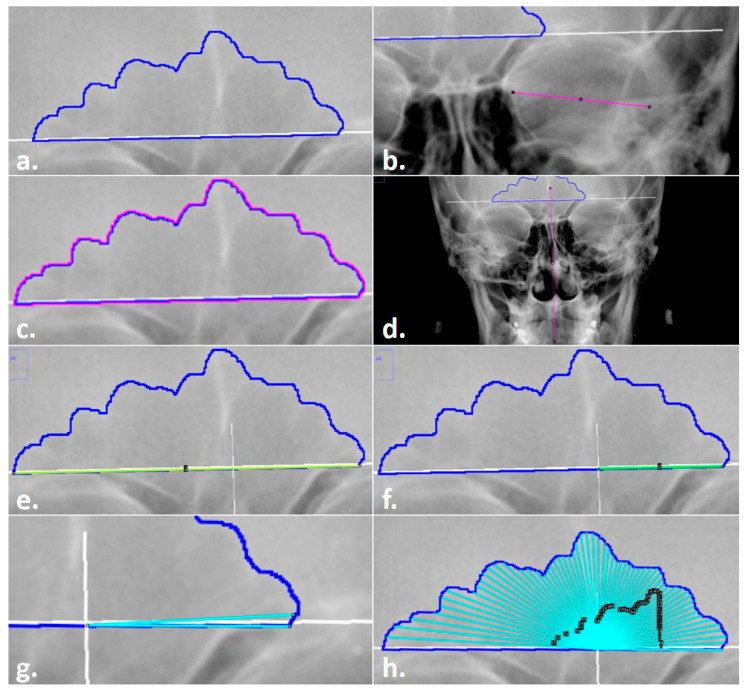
Steps in the Total Difference Method: (a) sinus outline on a radiograph; (b) measurement of orbital breadth for scaling; (c) highlighted sinus outline to be measured for the area and perimeter; (d) line drawn through midline landmarks to identify the origin of the sinus baseline; (e) line drawn for the baseline measurement; (f) line drawn from the origin for the right (side of the image) baseline measurement; (g) preliminary angle drawn at 3°; (h) completed angles with black ROI Manager numbered labels for editing, if required.
